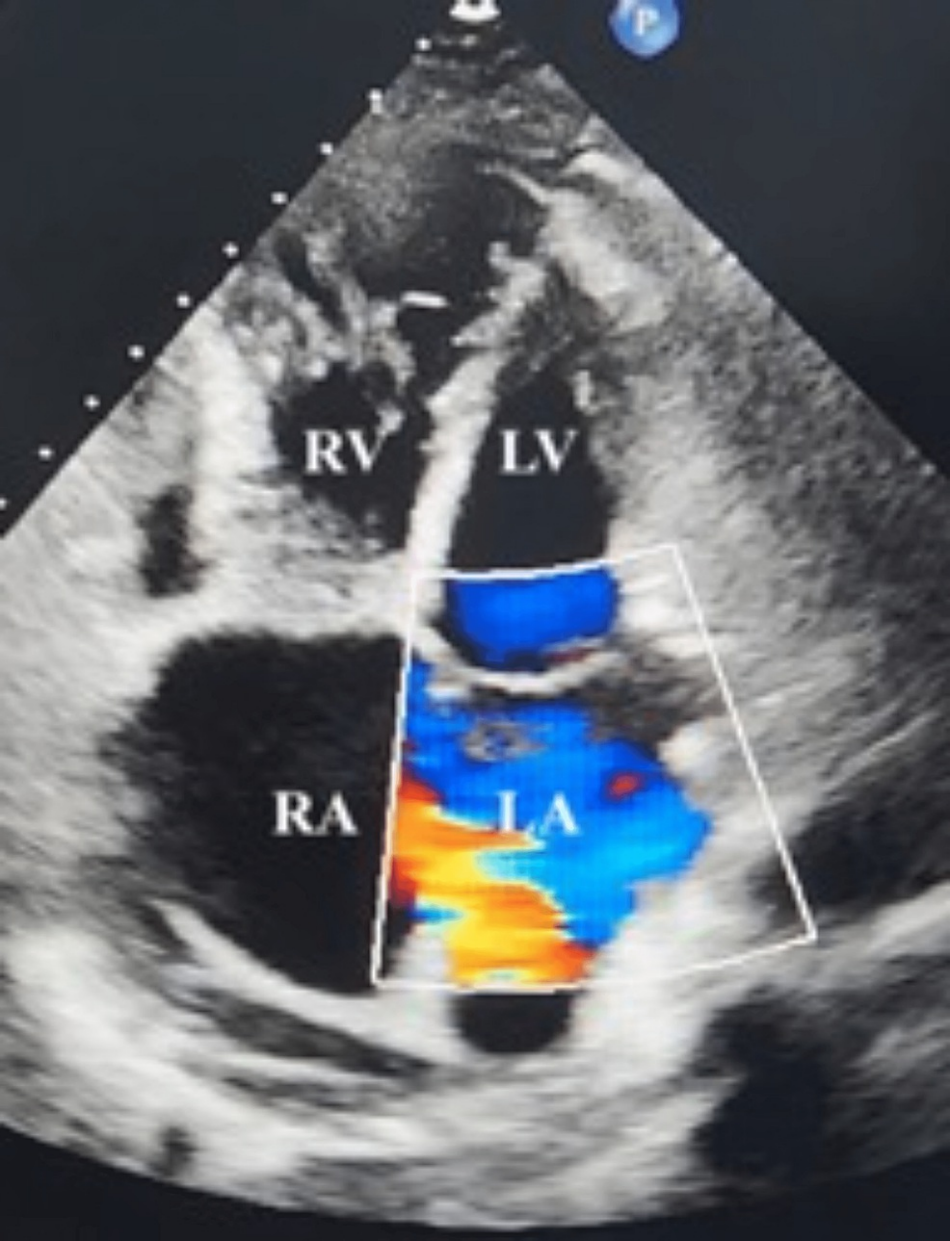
Pre-closure echocardiogram This is a four-chamber view showing the atrial septal defect (ASD) with color flow jet. LA, left atrium; LV, left ventricle; RA, right atrium; RV, right ventricle.
